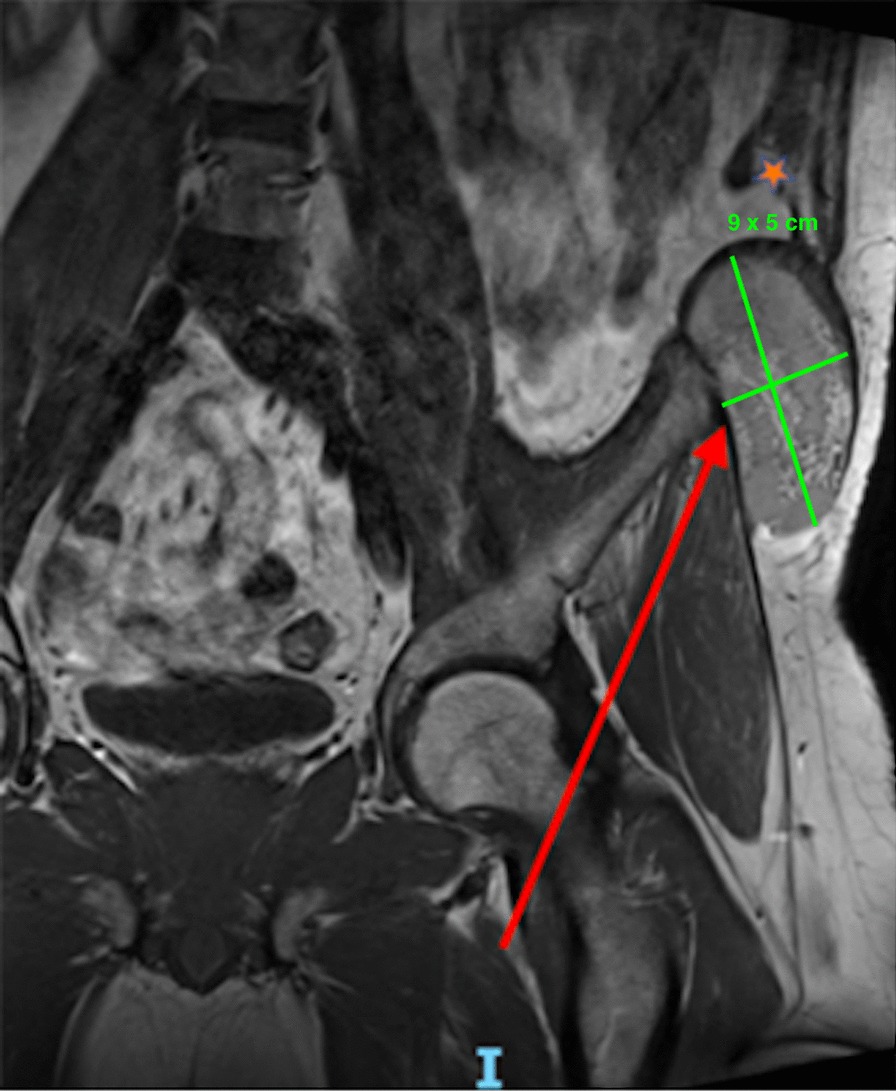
Coronal MRI views in T2 signal: red arrow: heterogeneous parietal collection, 9 × 5 cm; Orange star: internal oblique’s free edge, 7 cm from the iliac crest
